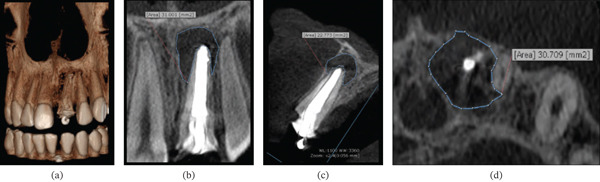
Diagnostic examination and planning of paraendodontic surgery using cone beam computed tomography. (a) 3D reconstructions, (b) coronal, (c) sagittal, and (d) axial views showing the quality of previous endodontic treatment, the location of the periapical lesion at the apical level, and the extent of the lesion, highlighting the breach of the vestibular bone plate.
